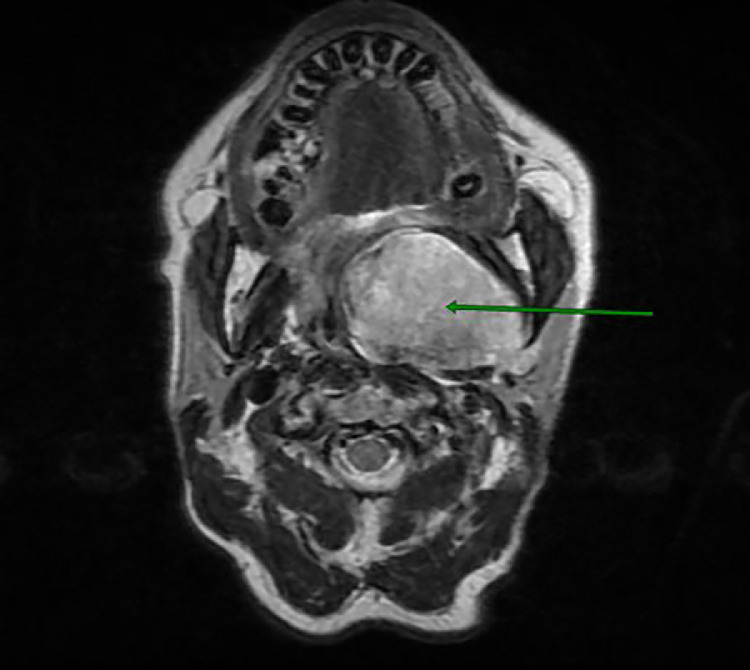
Axial T2-weighted image showing a hyperintense lesion in the left parapharyngeal space .
